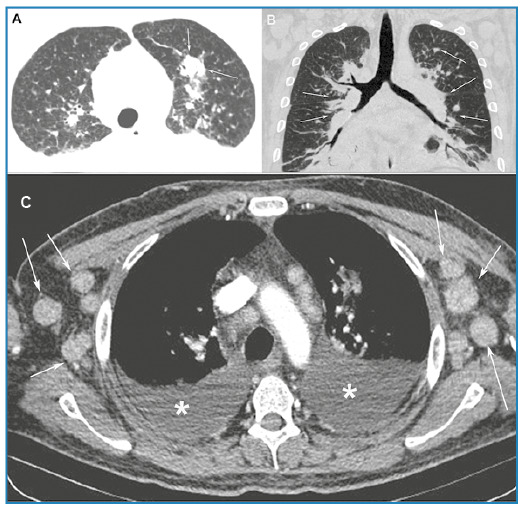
Computed tomography (CT) images of the chest with axial (A) and coronal (B) reconstructions showing an irregular parenchymal nodule (arrows in A) and thickening of the peribronchovascular interstitium and interlobular septa (arrows in B). C. Axial CT image showing axillary lymph-node enlargement (arrows) and bilateral pleural effusions (asterisks).
